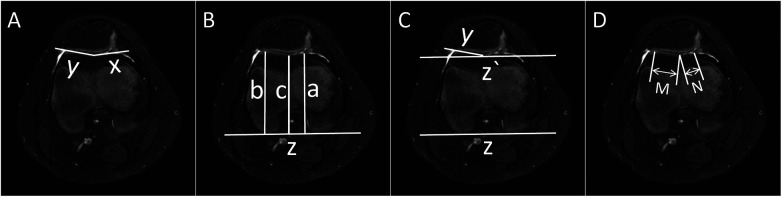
MRI measurements of femoral trochlear morphological parameters. (A) SA was defined as the angle between Line x and y, where Line x passed through the highest point of the medial condyle and the lowest point of the trochlear sulcus, and Line y passed through the highest point of the lateral condyle and the lowest point of the trochlear sulcus. (B) RTD was defined as the ratio of trochlear sulcus depth to the maximum anteroposterior diameter of the lateral femoral condyle, which was calculated by the formula [(a + b)/2−c]/b. (C) LTI was defined as the angle between Line y and Line z′; Line z′ was parallel to the posterior condylar tangent (Line z) and passes through the lowest point of the trochlear sulcus. (D) TFA was defined as the length ratio of the medial trochlear facet to the lateral trochlear facet, which was calculated as N/M.
